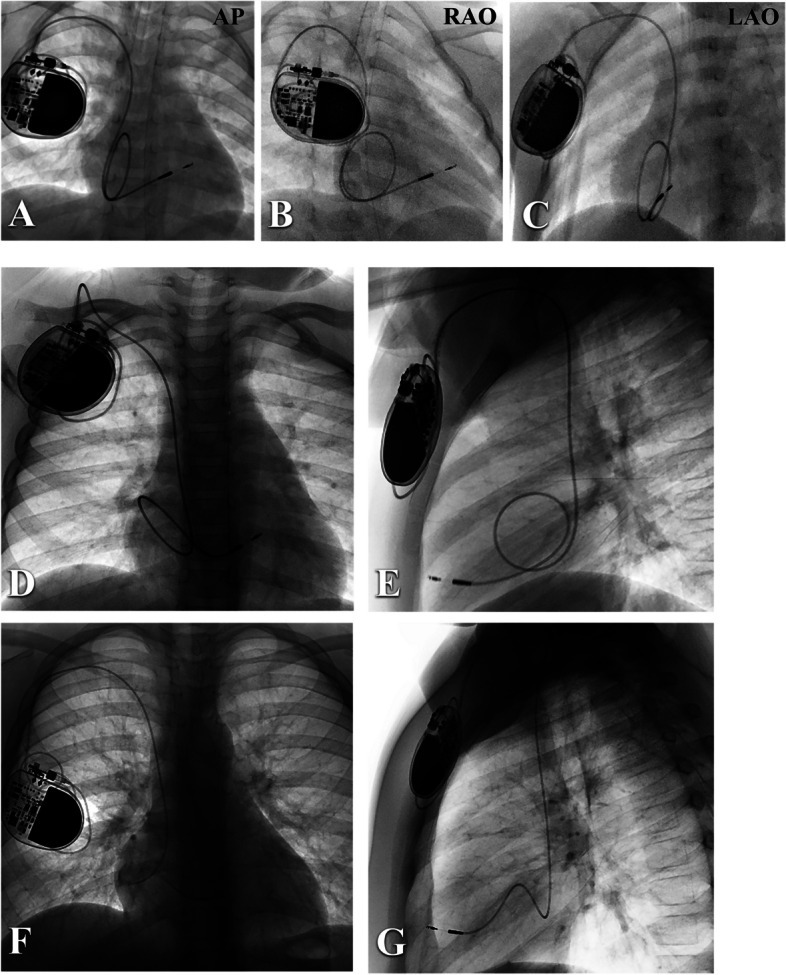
Radiology of the pacemaker implantation and follow-up. A, B and C are the X-ray images obtained during pacemaker implantation from AP, RAO, and LAO views. The 3830 lead was made into a redundant loop for a reservation of the growth in the RA. D and E are the anteroposterior and lateral chest radiographs at a 3-year follow-up. F and G are the anteroposterior and lateral chest radiographs in 5-year follow-up. The length of lead reserved in the RA is decreasing (AP: anteroposterior, RAO: right anterior oblique, LAO: left anterior oblique)
